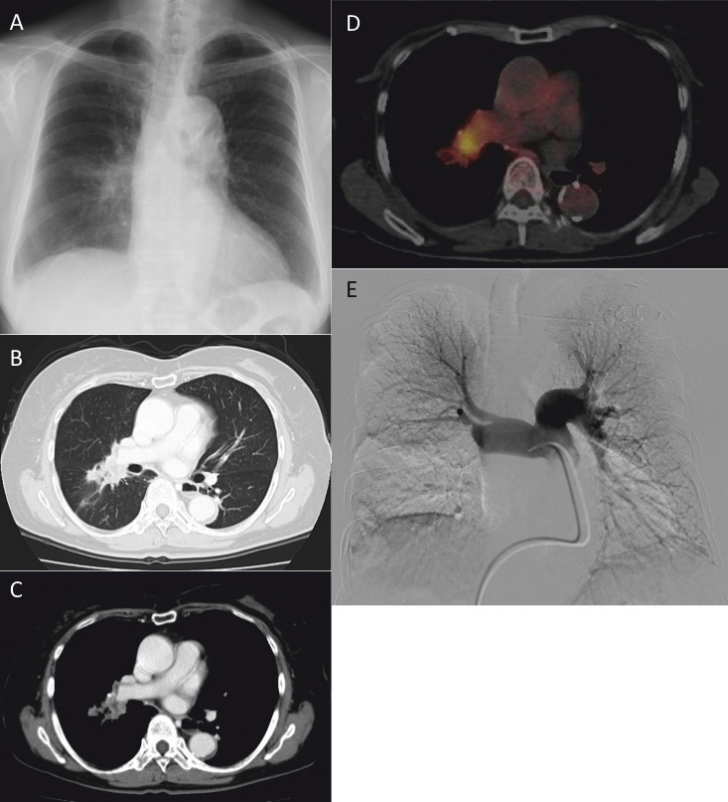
Radiological findings. A: Chest X-ray showed a mass shadow in the right middle lung field. B, C: Chest CT showed a tumor with an irregular border centered in the hilar region extending from S6 to the middle lobe of the right lung. Stenosis and occlusion of the pulmonary artery were found on mediastinal window setting. D: Positron emission tomography showed fluorodeoxyglucose uptake in the tumor. E: Angiography showed complete occlusion of the pulmonary trunk at the periphery proximal to the bifurcation of the posterior ascending branch, and a filling defect at the root of this branch.
